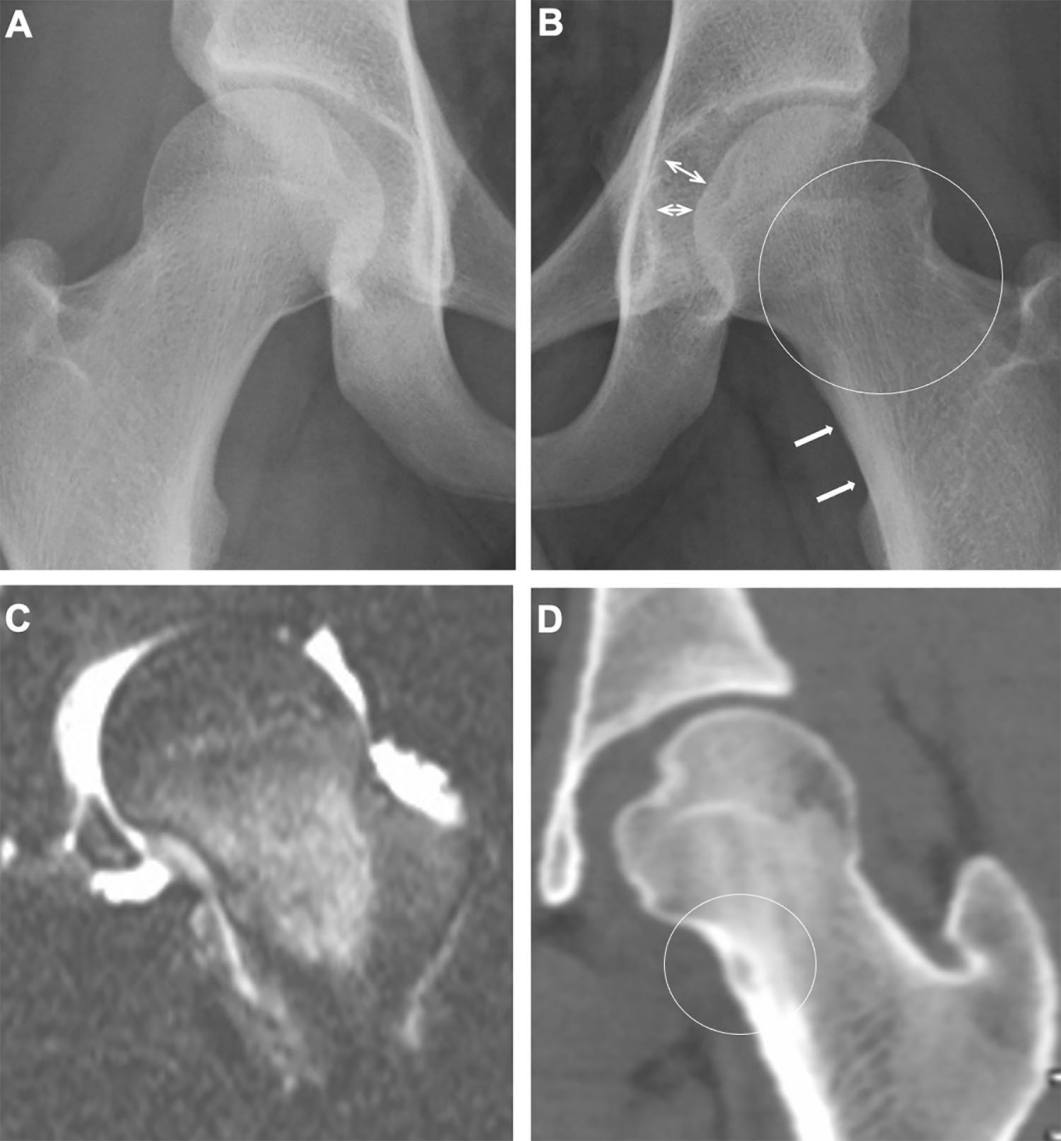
Intraarticular osteoid osteoma. A–D. A 17-year-old boy with painful left hip. The frontal radiographs of the hips showed mild hyperostosis of the medial cortex (arrows, B) and osteopenia of the left femoral neck (circle, B) and mildly increased acetabulo-femoral distance (double headed arrows, B) and a normal right hip (A). Fat-suppressed T2-weighted coronal image showed bone marrow edema of the femoral neck along with increased joint fluid and irregular synovial surfaces (C). CT revealed a small nidus in the osteosclerotic medial cortex of the femoral neck (circle, D)
